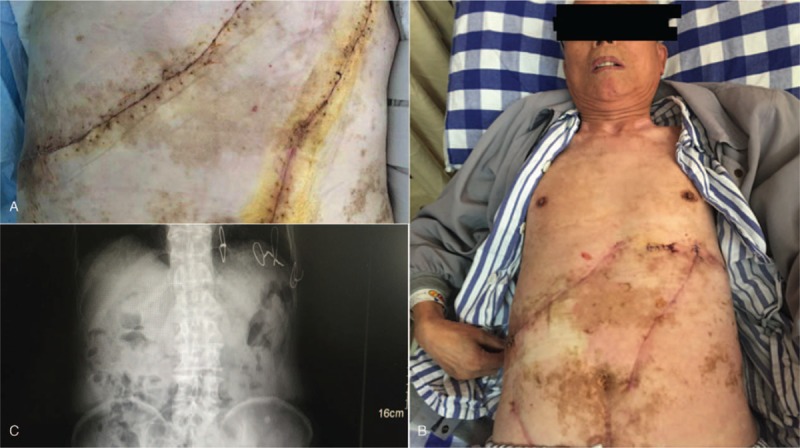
(A and B) Postoperative follow-up 3 months after surgery showed good healing; (C) X-ray radiography shows that the ribs were healing well.
